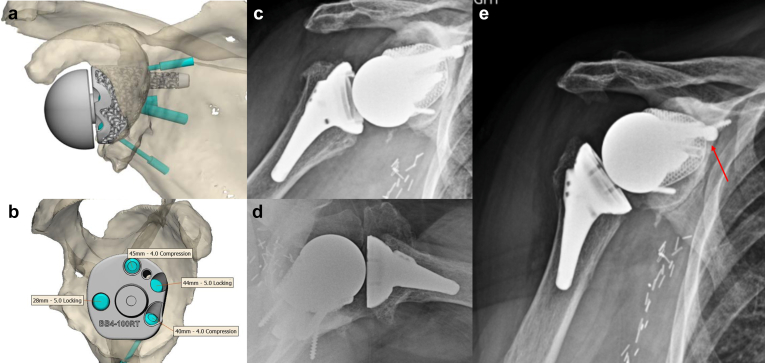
(a) Pre-operative plan demonstrating glenosphere placement and final implant construct. (b) Pre-operative plan showing screw configuration. (c) Immediate post-operative anteroposterior (AP) radiograph. (d) Immediate post-operative axillary radiograph (alternate view). (e) 12-month post-operative AP radiograph demonstrating a broken post (red *arrow*).
